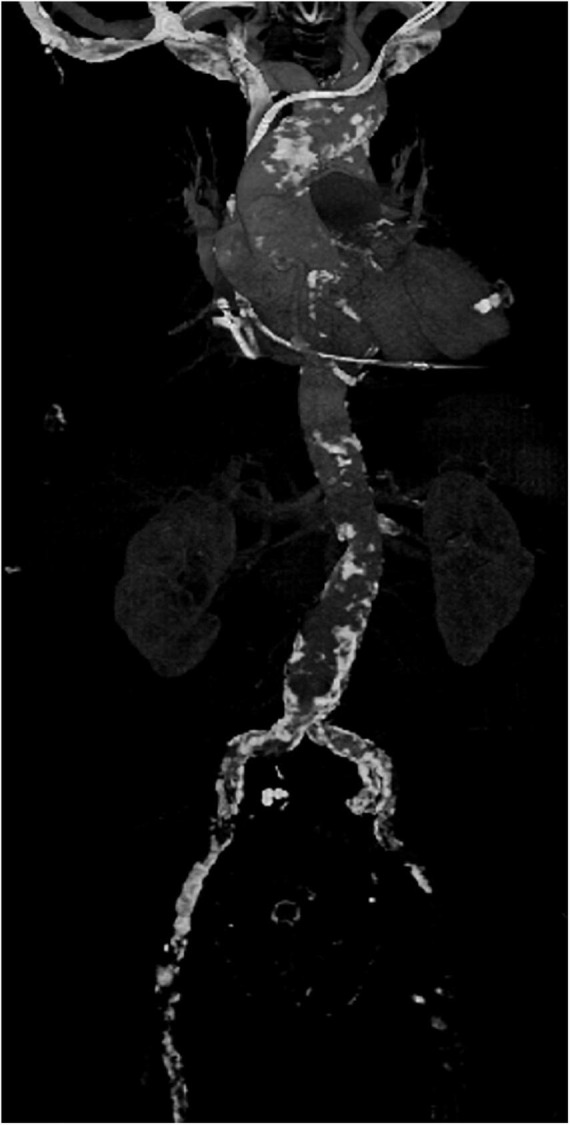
Example of a computed tomography (CT) scan of the aorta of a patient with severe arteriosclerosis in the axillary artery, aortic arch abdominal aorta and especially the femoral vessels in which minimally-invasive surgery was performed by using an antegrade arterial perfusion *via* arteria axillaris.
